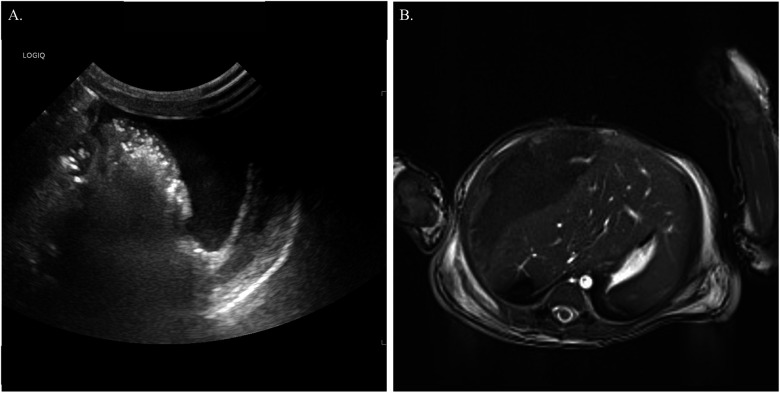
(A) Ultrasound abdomen: a single sonographic image of the left lower quadrant demonstrates a moderate amount of mildly complex fluid surrounding partially visualized bowel loops. (B) MRI liver: a single axial T2-weighted image of the upper abdomen demonstrates a moderate amount of heterogenous, predominantly hypointense fluid within the subcapsular anterolateral right hepatic lobe. The subcapsular hematoma exerts a moderate mass effect on the liver, with midline hepatic shift toward the left abdomen/left upper abdominal quadrant.
